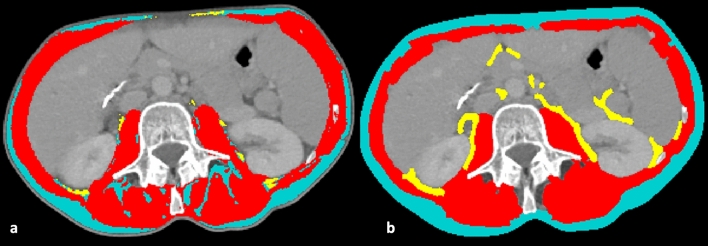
Axial CT image at the level of the third lumbar vertebra (L3), showing segmentation performed by the automatic tool a. Since the visceral adipose tissue (yellow) and the skeletal muscle area (red) on the abdominal wall were considered not properly segmented, in this case a semi-automatic segmentation b was favored
